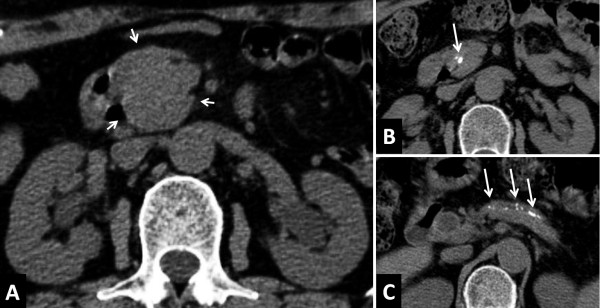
CT findings in a 67-year-old woman with pancreatic head swelling. (A) CT at diagnosis in May 2005 showing pancreatic head swelling (arrows). (B), (C) CT 27 months later in August 2007 showing pancreatic stone formation and pancreatic atrophy (arrows). Data are reprinted from Ref. [41] with permission from the Journal of Gastroenterology.
